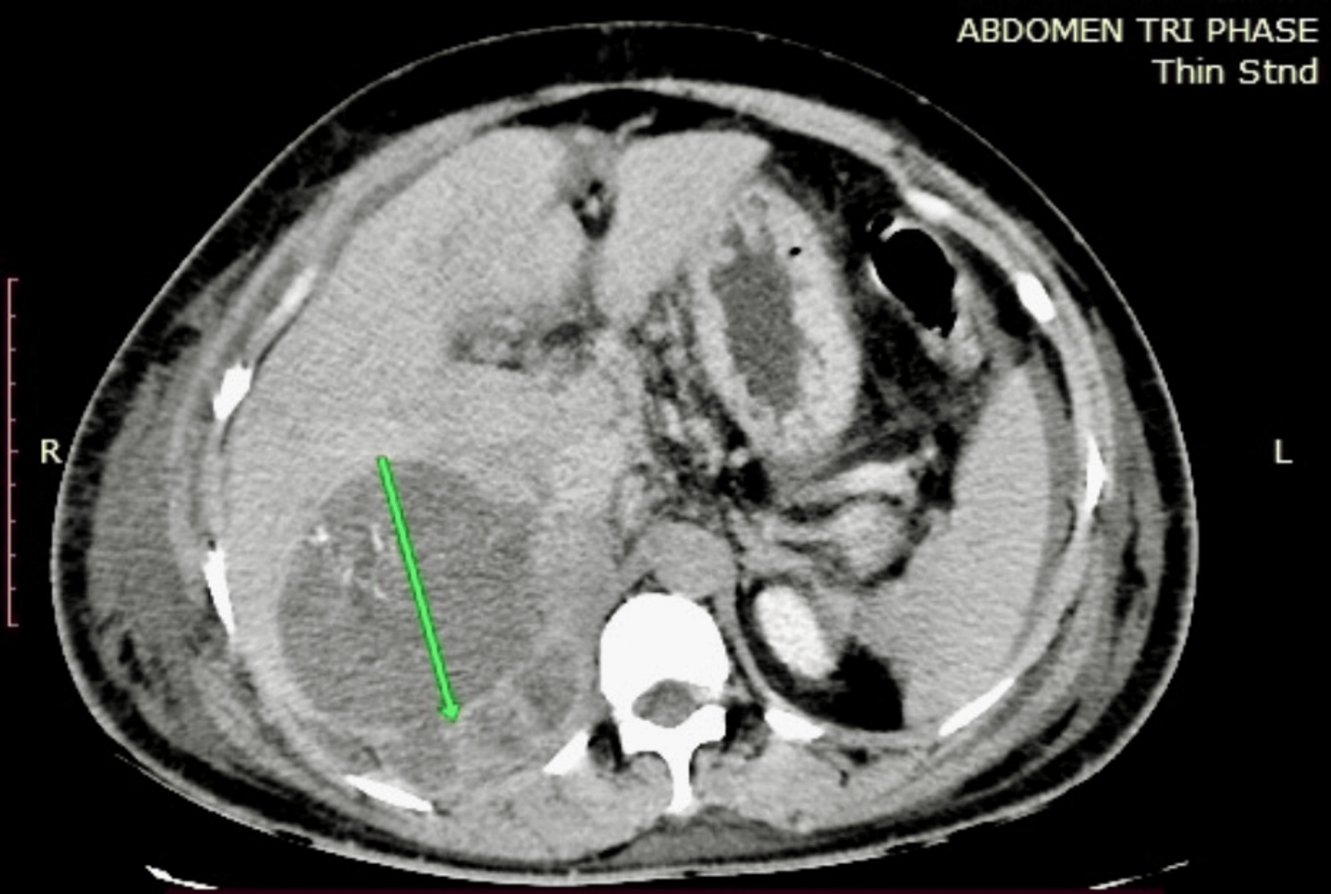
Coronal section of contrast-enhanced computed tomography (CECT) showing daughter cysts (arrow) within a primary hydatid cyst.
